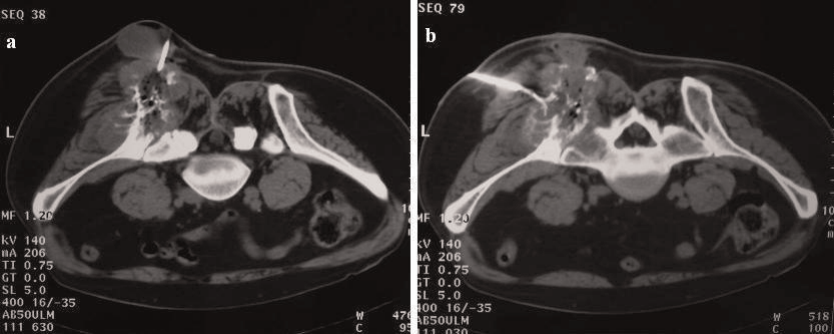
Unenhanced CT scans obtained during ablation show the large exophytic ileac mass (A, B). The radio frequency needle inserted into the mass under CT guidance through posterior approach.
